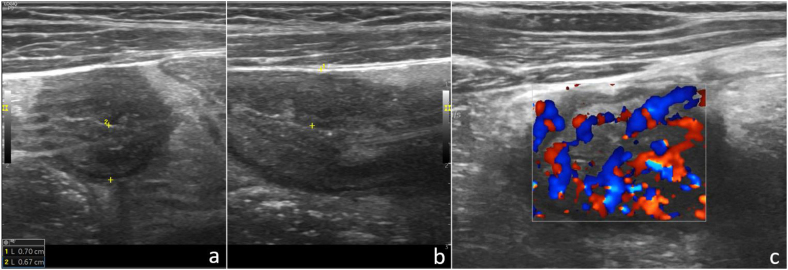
Corte longitudinal (a) y transversal (b) con sonda lineal de alta frecuencia (8-12 MHz) a nivel de fosa iliaca derecha donde se identifica el íleon terminal con engrosamiento generalizado de su pared y cuantificación de su espesor. c) Imagen similar a la primera donde se evidencia un aumento de la vascularización del íleon mediante Doppler color.
